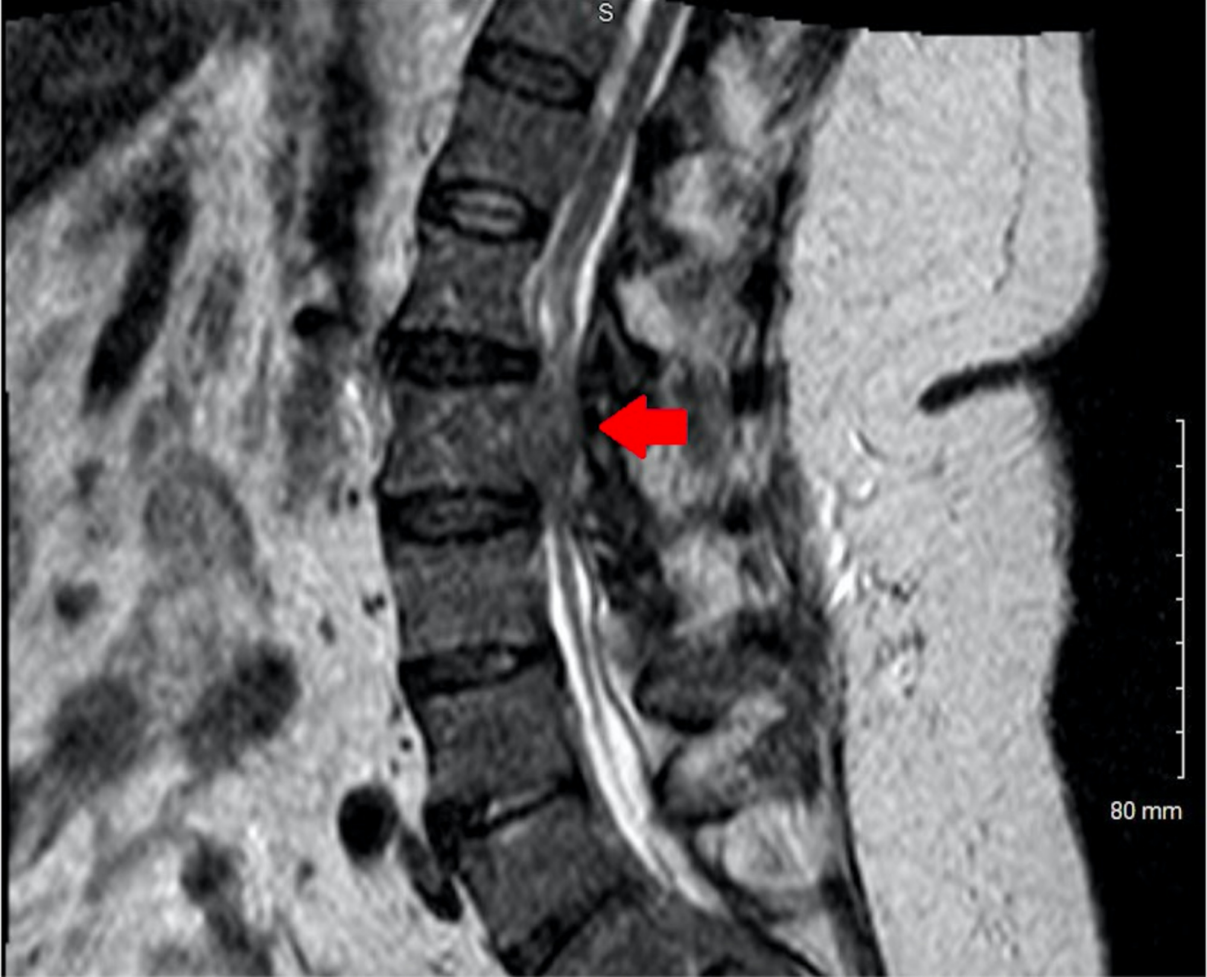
MRI lumbar spine - lesion (red arrow) at L2 level causing compression of cauda equina.
